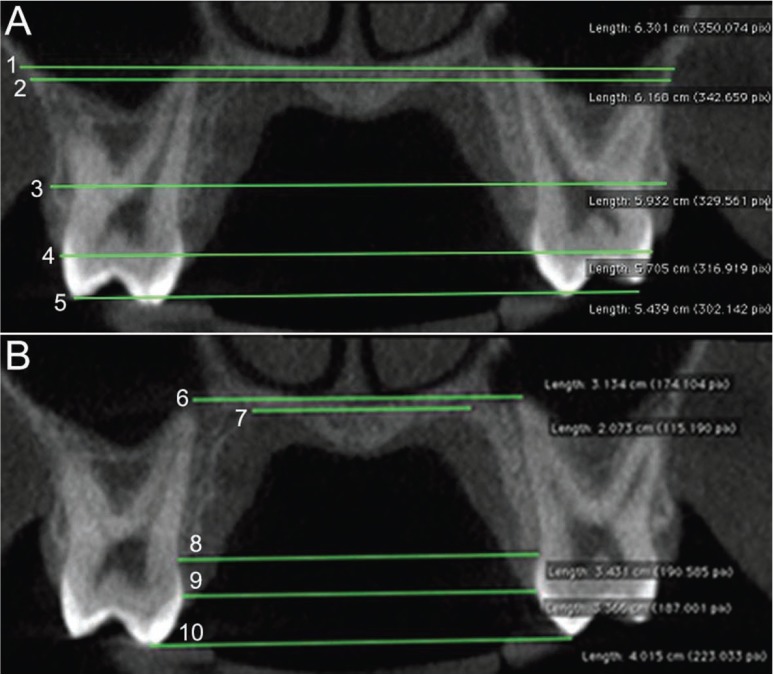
External (A) and internal (B) measurements (n=10) taken through ST-CBCT in each patient to analyze the skeletal effects of rapid maxillary expansion. Legend: Measurements from 1 to 10 correspond, respectively, to the skeletal parameters NF, HP, BAC, DA.E’, DA.E, HP’, PA, LAC, DA.I’ and DA.I. Additional description of these parameters is found in  Table 1.
